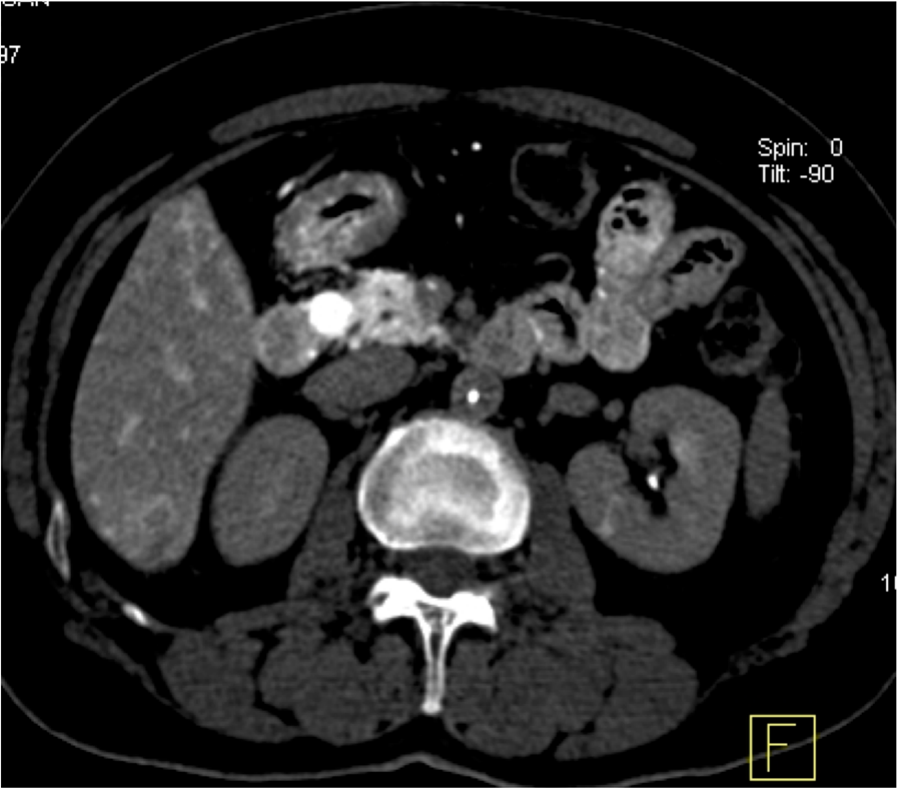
CT during celiac artery angiography shows an insulinoma in the pancreas head
